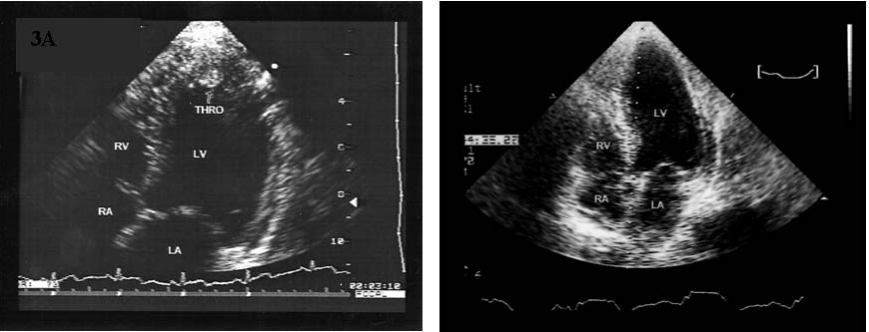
A. Apical four chamber view. It depicts a thrombus in the apex (arrows) Abbreviations: LV:left ventricle, RV: right ventricle, LA: left atrium, RA: right atrium, THRO: thrombus. B. The thrombus has disappeared after anticoagulant therapy. Abbreviations: as in figure 3A.
